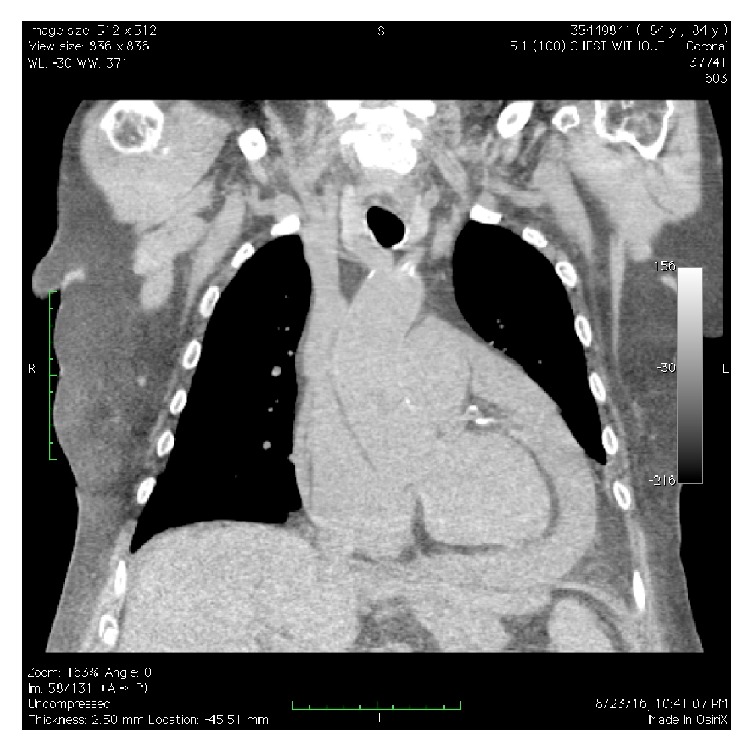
Computerized tomography (CT) of the chest showing new pericardial effusion and small bilateral pleural effusions with adjacent atelectasis when compared to the CT of the chest performed 21 days priorly.
